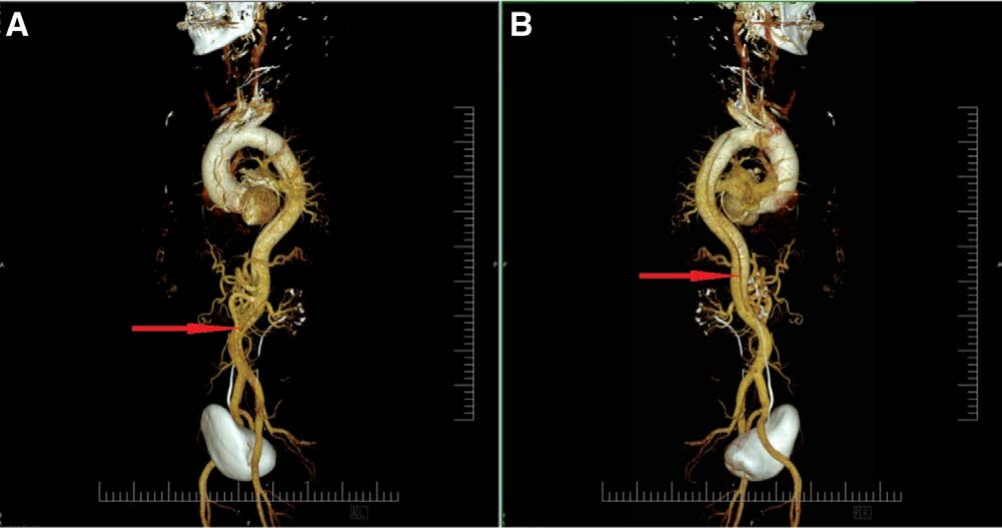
VRT of CT angiography. Stanford type A aortic dissection with an intimal flap and entry tear (yellow arrow) originating in the region of the right coronary sinus (A). The dissection extends through the aortic arch, descending thoracic aorta, and abdominal aorta, reaching the iliac arteries (blue arrow – dissection of abdominal aorta) with a reentry point in the common femoral artery (not shown). Propagation of the dissection flap into the aortic arch branches, with involvement of the left subclavian artery (B). CT = computed tomography, VRT = volume-rendered 3D reconstruction.
